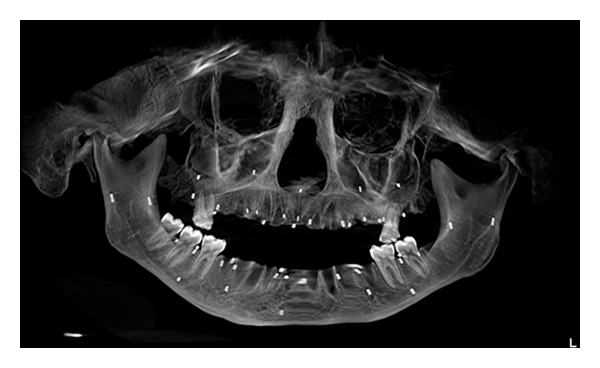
Panoramic view of the skull radiograph with 10 degree left tilt. Opaque gutta-percha markers are observed in the image.
